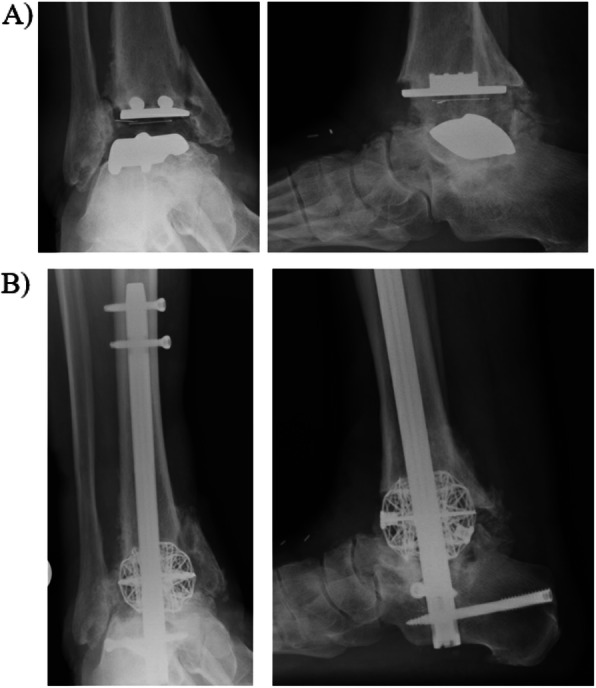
Tibiotalocalcaneal (TTC) arthrodesis for case of failed total ankle arthroplasty. a AP and lateral radiographs demonstrate STAR ankle prosthesis with evidence of talar component collapse with erosion into subtalar joint. Medial malleolus fracture present as well. b Patient underwent TTC arthrodesis with 3D titanium cage. The cage is packed with allograft/autograft to enhance healing
